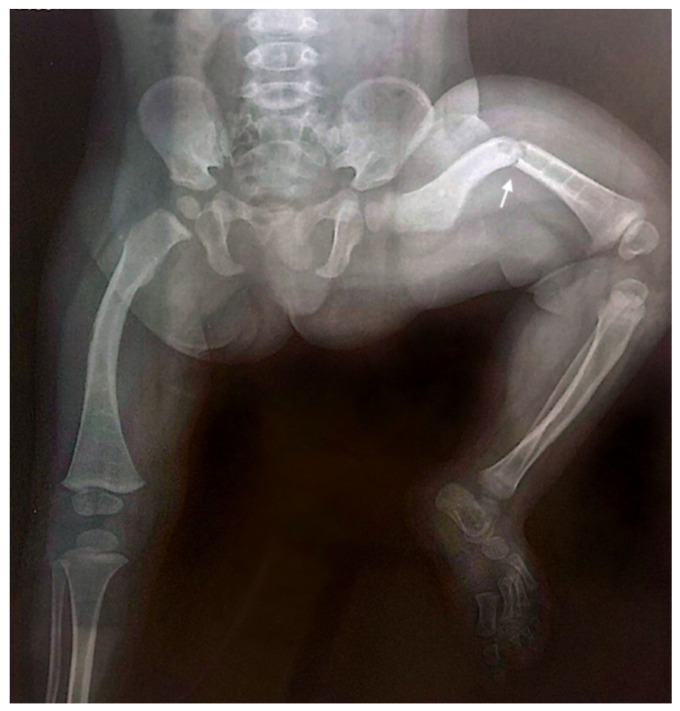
Anteroposterior radiograph of the lower limbs taken at the age of one year and seven months, showing poor consolidation of the left femoral fracture (indicated by the arrow), characterized by persistent displacement and inadequate callus formation. Generalized osteopenia is also observed, with diffusely reduced bone density consistent with the underlying condition.
